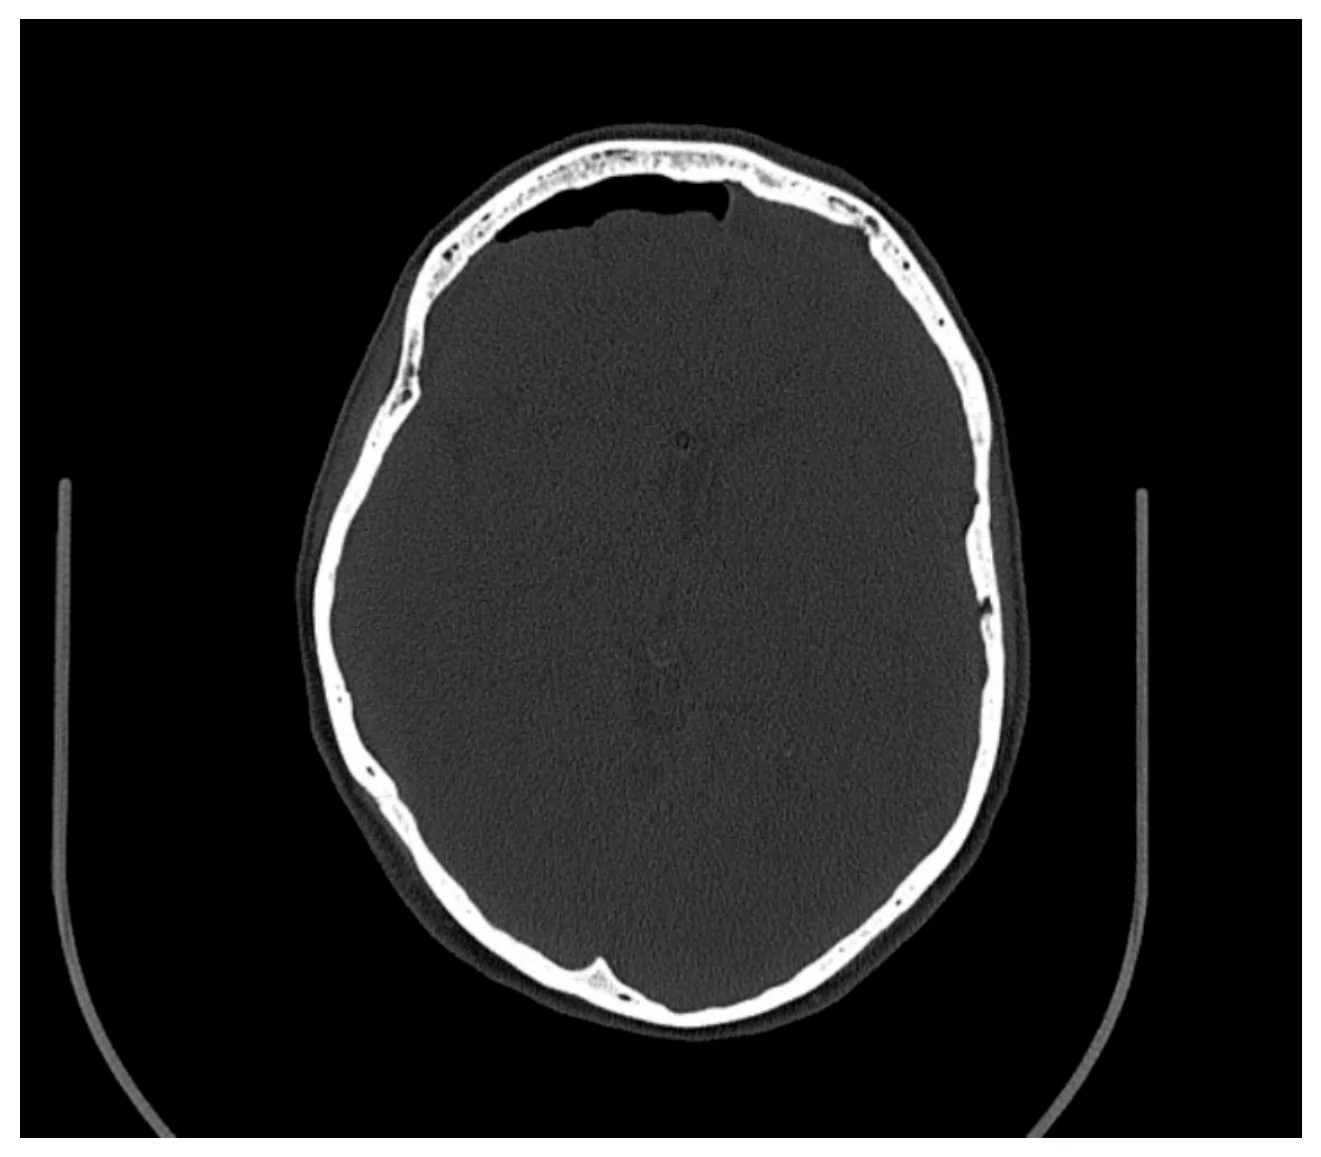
Preoperative CT scan demonstrating intracranial gas collections (pneumocephalus).
